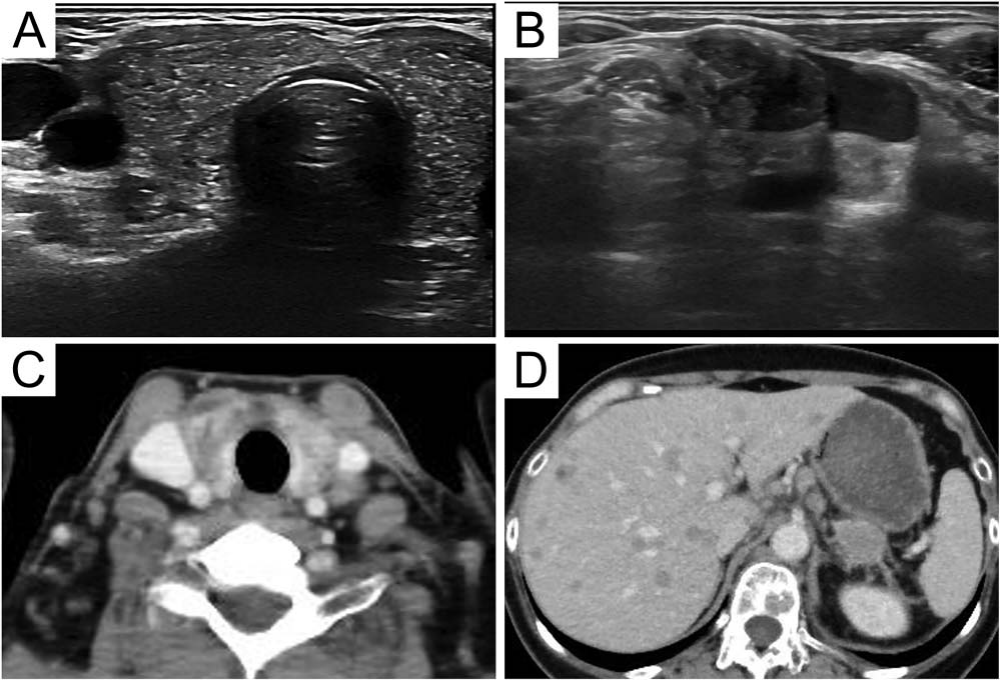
Imaging findings. (A) Ultrasonographic image showing scattered dot-like high
